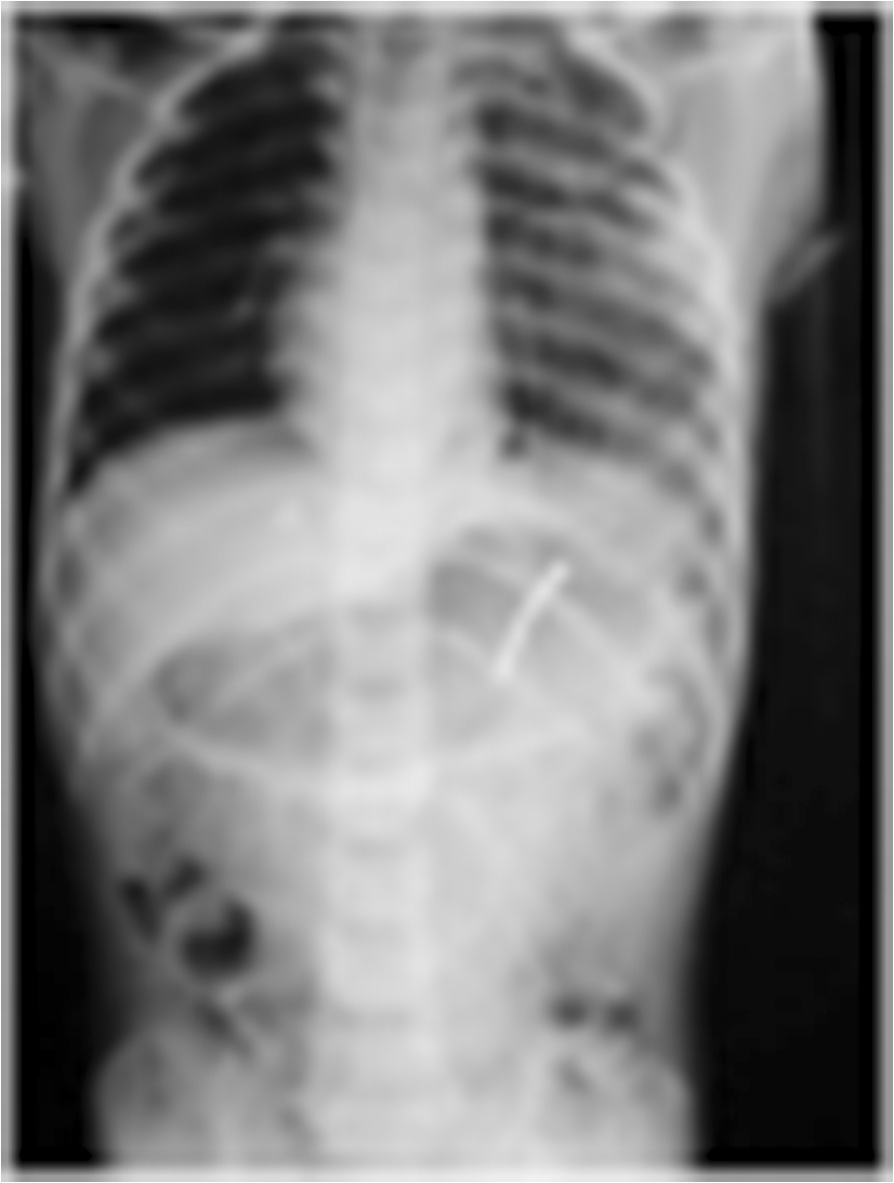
Chest radiograph at the time of the patient’s hospital admission. Left lung middle lobe is contused (hematoma formation), with obliteration left costo-phrenic angle. The bamboo stake is barely discernible by faint translucent lines.
